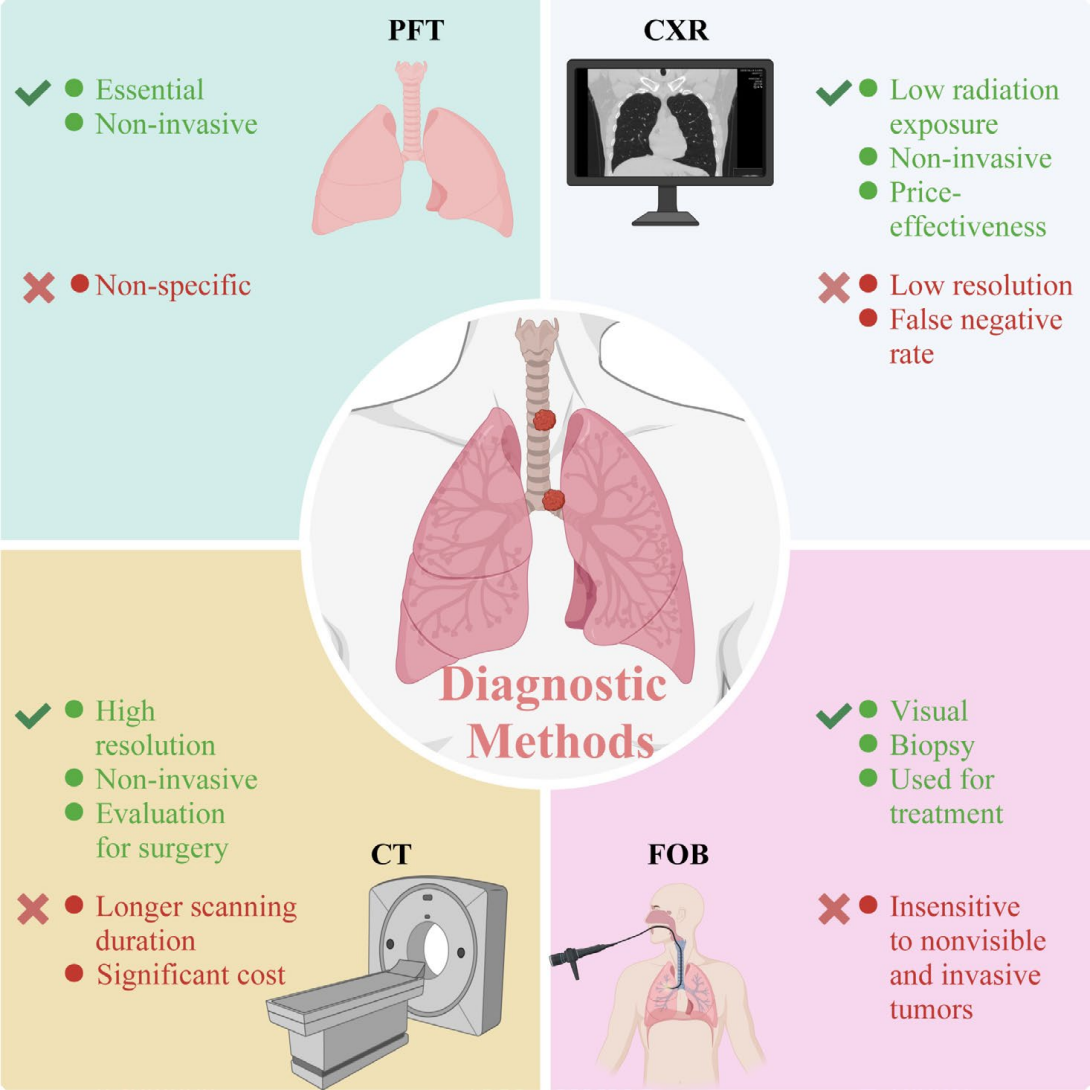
Overview of diagnostic methods for PTBTs. Diagnostic methods for PTBTs mainly include pulmonary function tests (PFTs), chest radiography (CXR), computed tomography (CT), and fiberoptic bronchoscopy (FOB).
